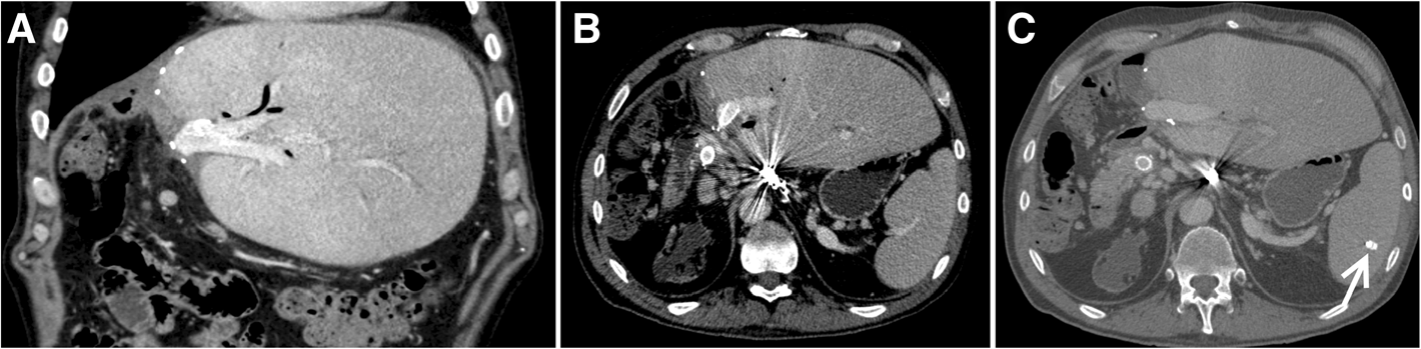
Contrast-enhanced PET-CT of the abdomen 3 years post-interventional: A, B, C Coronal and axial venous phase images demonstrate a patent portal vein with stents in situ, a left hepatic lobe hypertrophy and the Amplatzer® vascular plug (marked with an arrow in C) within the spleen.
